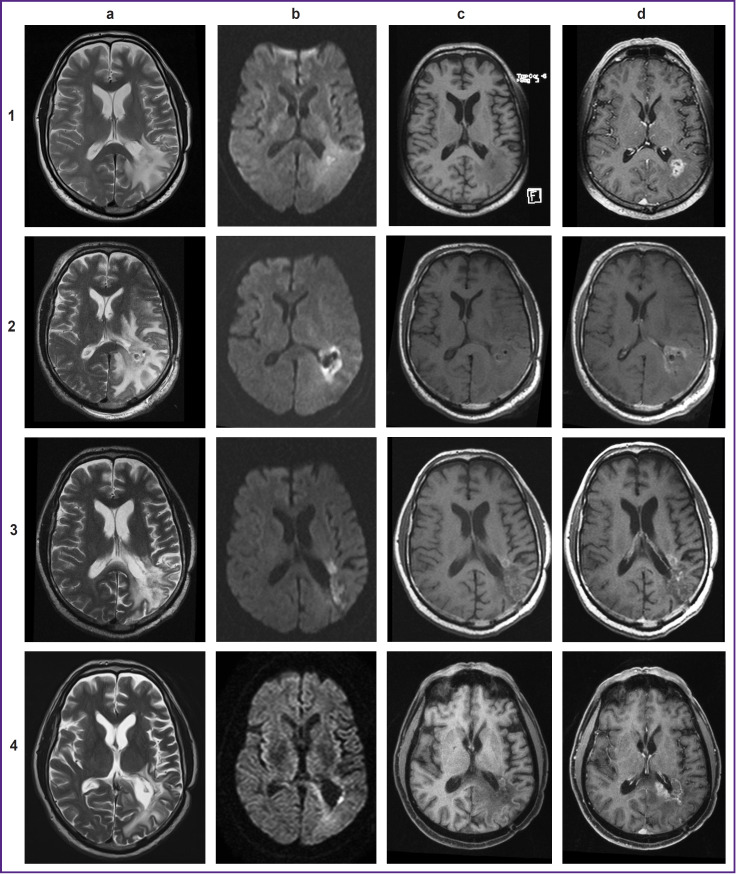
MRI of the brain with contrast intensifying: *1* — before intervention: (a) T2-weighted image (WI) before intervention; (b) diffusion-weighted images (DWI) (b=1000); (c) T1-WI before contrast; (d) post-contrast T1-WIs; *2* — after stereotactic photodynamic therapy: (a) T2-WI, increase in the perifocal area of edema; (b) DWI (b=1000), limitated diffusion in the affected area; (c) T1-WI before contrast, a minor increase in signal intensity in the affected area; (d) T1-WIs post-contrast, decreased accumulation of the contrast agent in the affected area; *3* — 6 months after stereotactic photodynamic therapy: (a) T2-WI, reduction in the area of perifocal edema; (b) DWI (b=1000), limitated diffusion in the affected area and anterior to it; (c) T1-WI before contrast, a minor hyperintense area anterior to the treatment area; (d) post-contrast T1-WIs, a minor area of insignificant accumulation of the contrast agent in the affected area; *4* — 14 months after stereotactic photodynamic therapy (continued growth): (a) T2-WI; (b) DWI (b=1000), remaining minor area of a limitated diffusion in the impact area; (c) T1-WI before contrast; (d) post-contrast T1-WIs, the affected area still has a insignificant accumulation of the contrast agent and a new area of accumulation of the contrast agent measuring 38×36×33 mm
